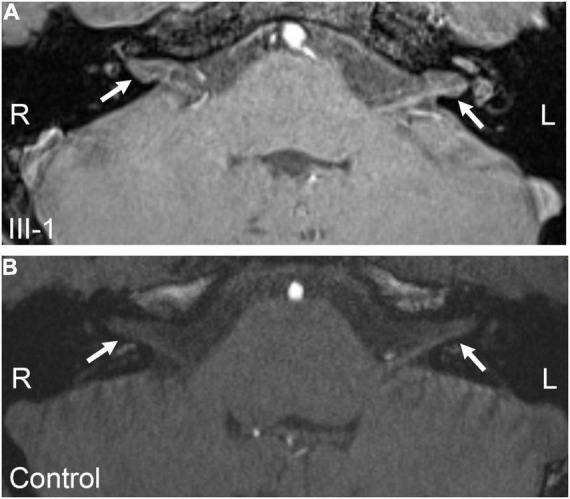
MRI of internal auditory canal. (A) Image of III-1. (B) Image of normal control. The arrows marked the auditory nerve. R, right; L, left.
